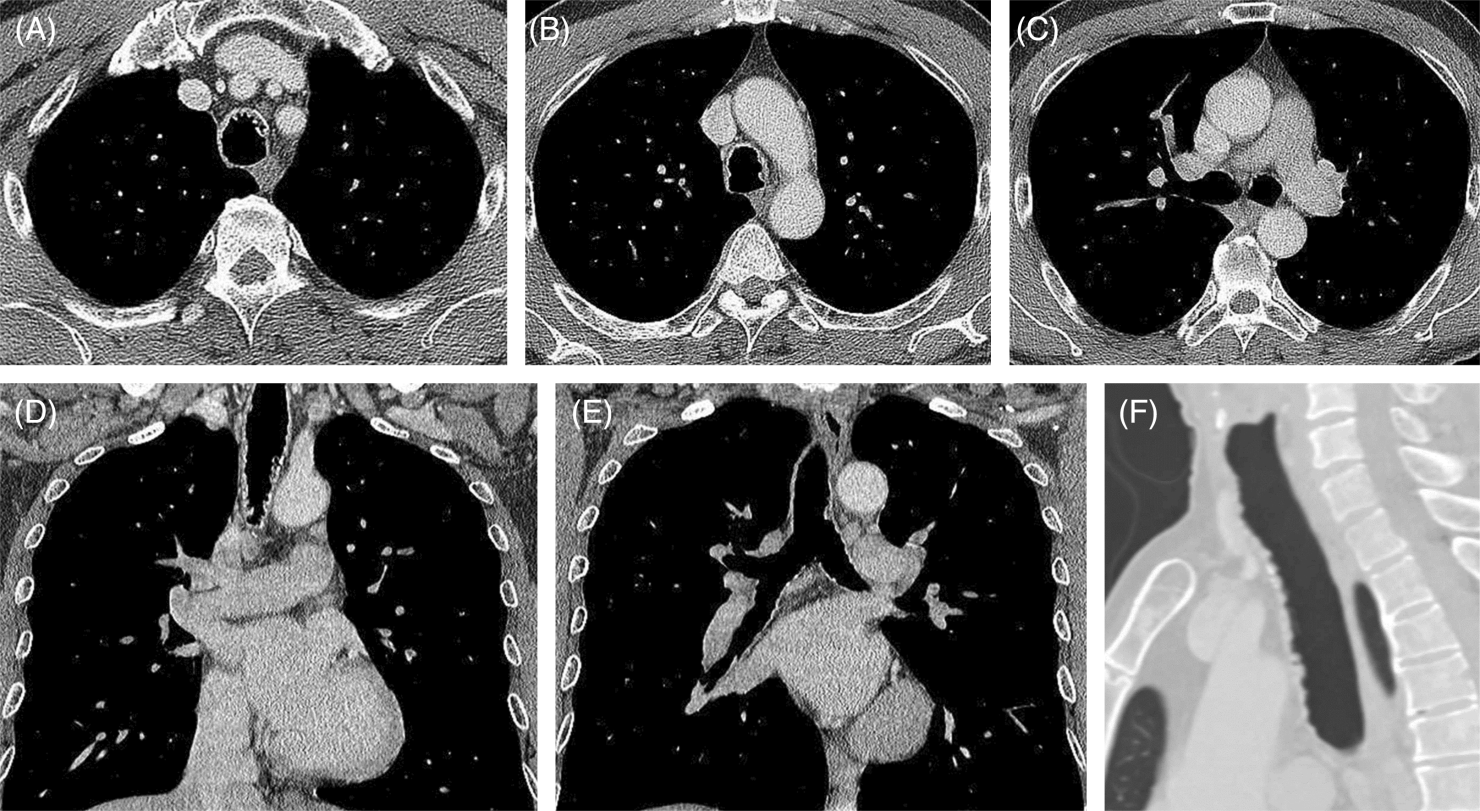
Thoracic CT images in transverse (A–C), coronal (D–E), and sagittal (F) views reveal multiple nodular ingrowths in the trachea, many of which exhibit mineral radiodensities. The posterior tracheal wall and the main bronchi are spared.
